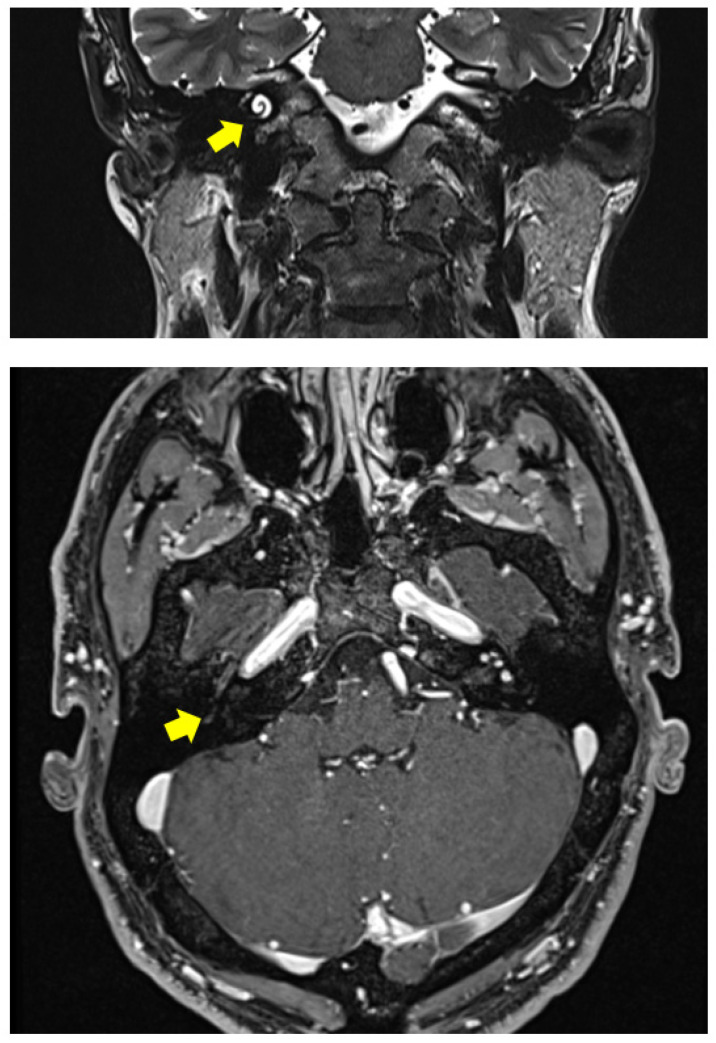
Clinical Case 1: 3 Tesla MRI with gadolinium showing an ILS filling the right cochlea with initial vestibular involvement.
